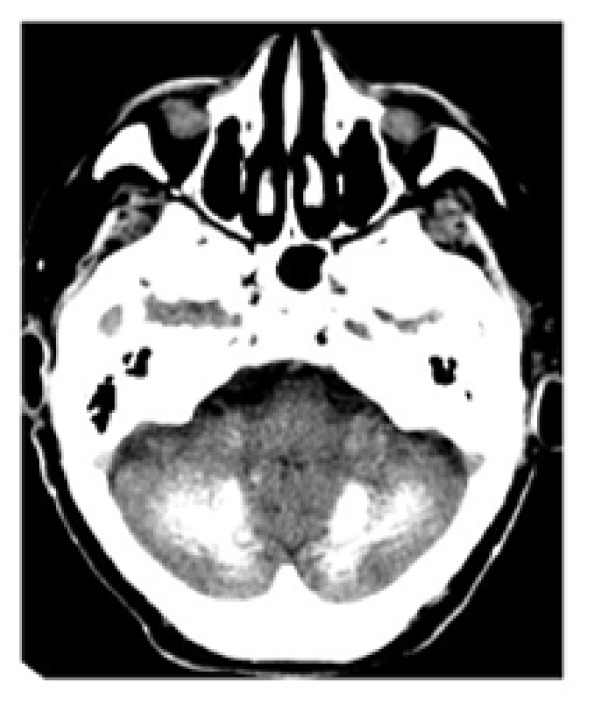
Cerebellar calcifications (CT scan).
